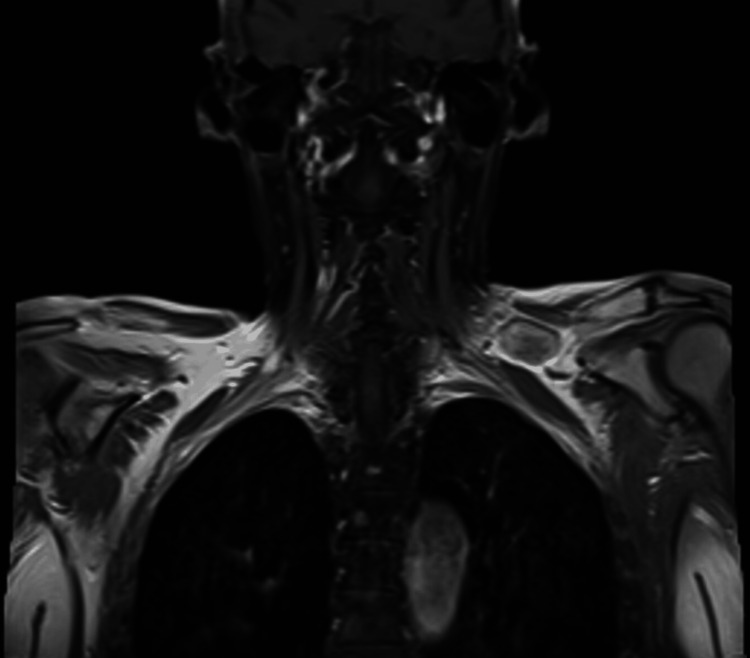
MRI of the brachial plexus, with a purple arrow indicating left supraclavicular adenopathy, showing no brachial plexus involvement. MRI: magnetic resonance imaging
